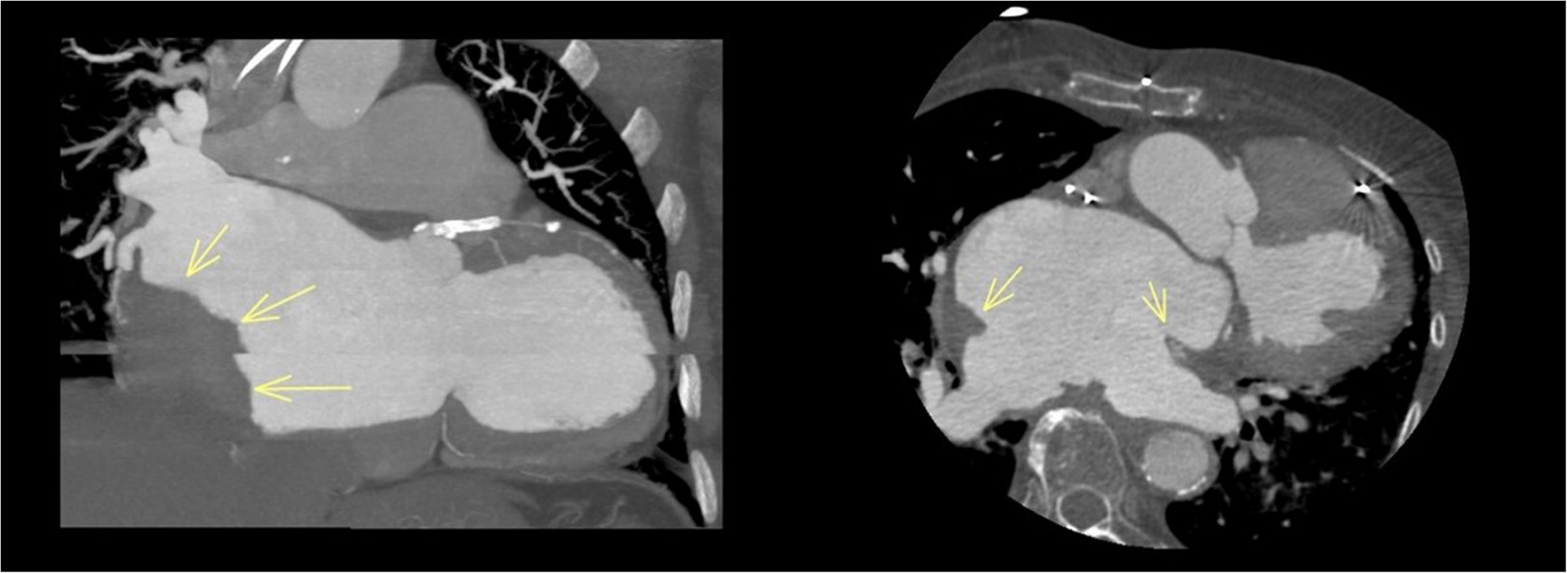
CT scan evidencing the thrombus in the neo-atrium
